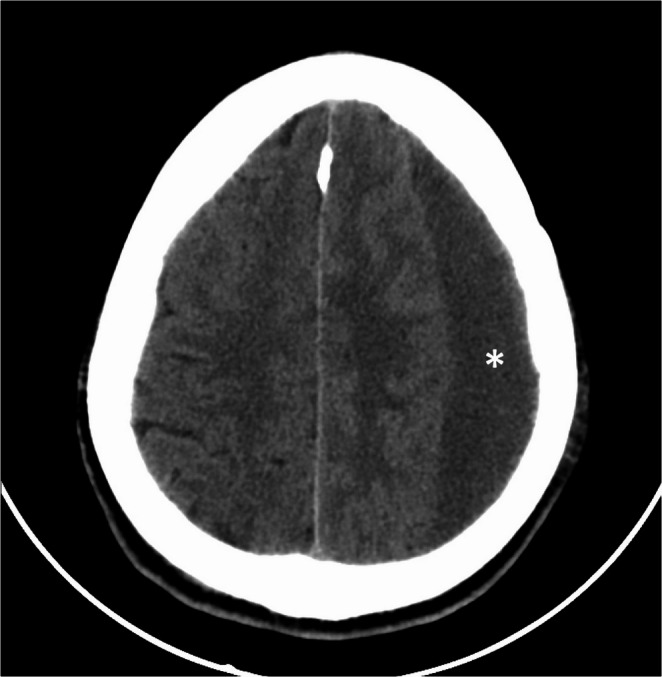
A 36-year-old male migrant, reported history of violence with head trauma. Axial CT of head: chronic subdural hematoma in the left hemisphere (asterisk)
